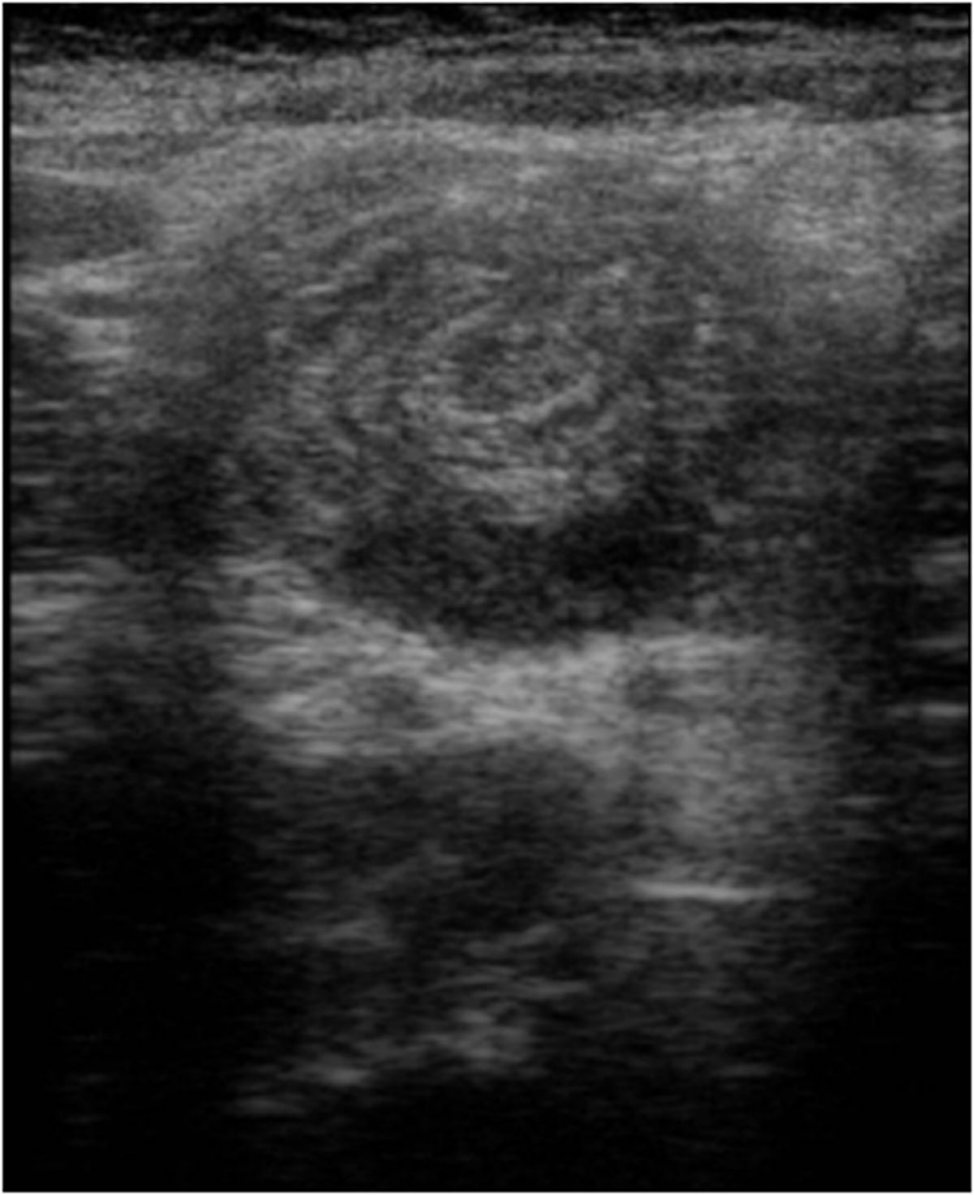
Ultrasound features of intussusceptions. US obtained at the apex shows a hyper echoic outer ring separated from a hypo echoic center by a thin hyper echoic ring, which likely represents the opposed serous surface of the intussuscepted.
